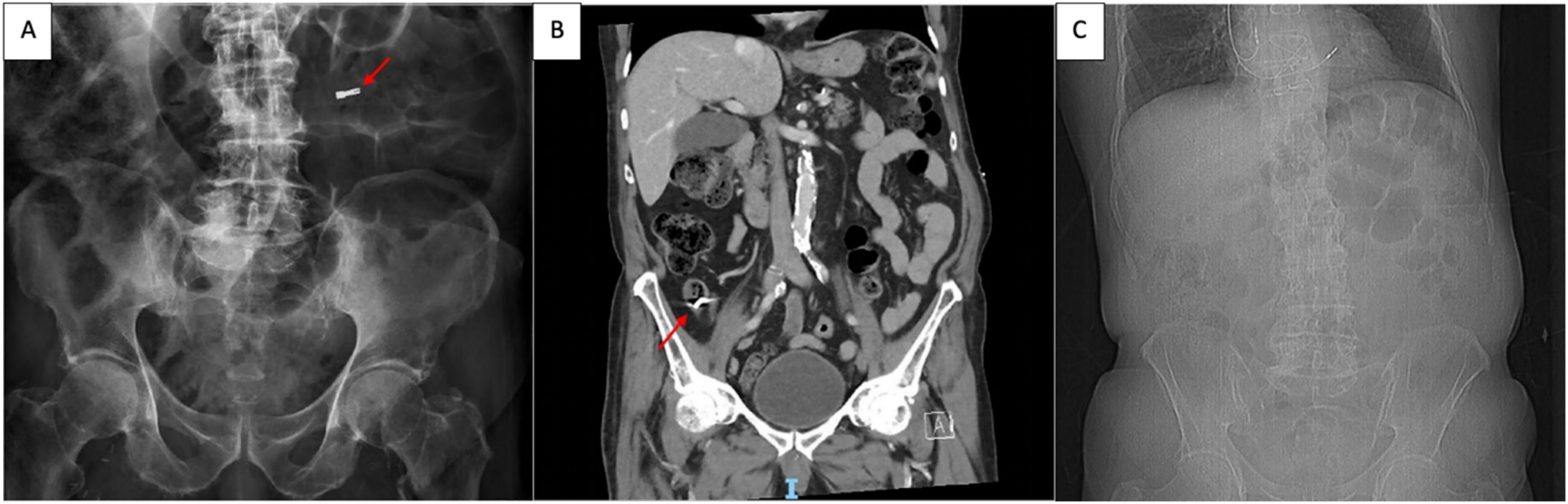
Abdominal phase: (A) Abdominal XR showing metallic foreign body in the abdomen → (B) CT abdomen showing impaction at ileo‐caecal valve → (C) CT scout confirming spontaneous passage of foreign body.
